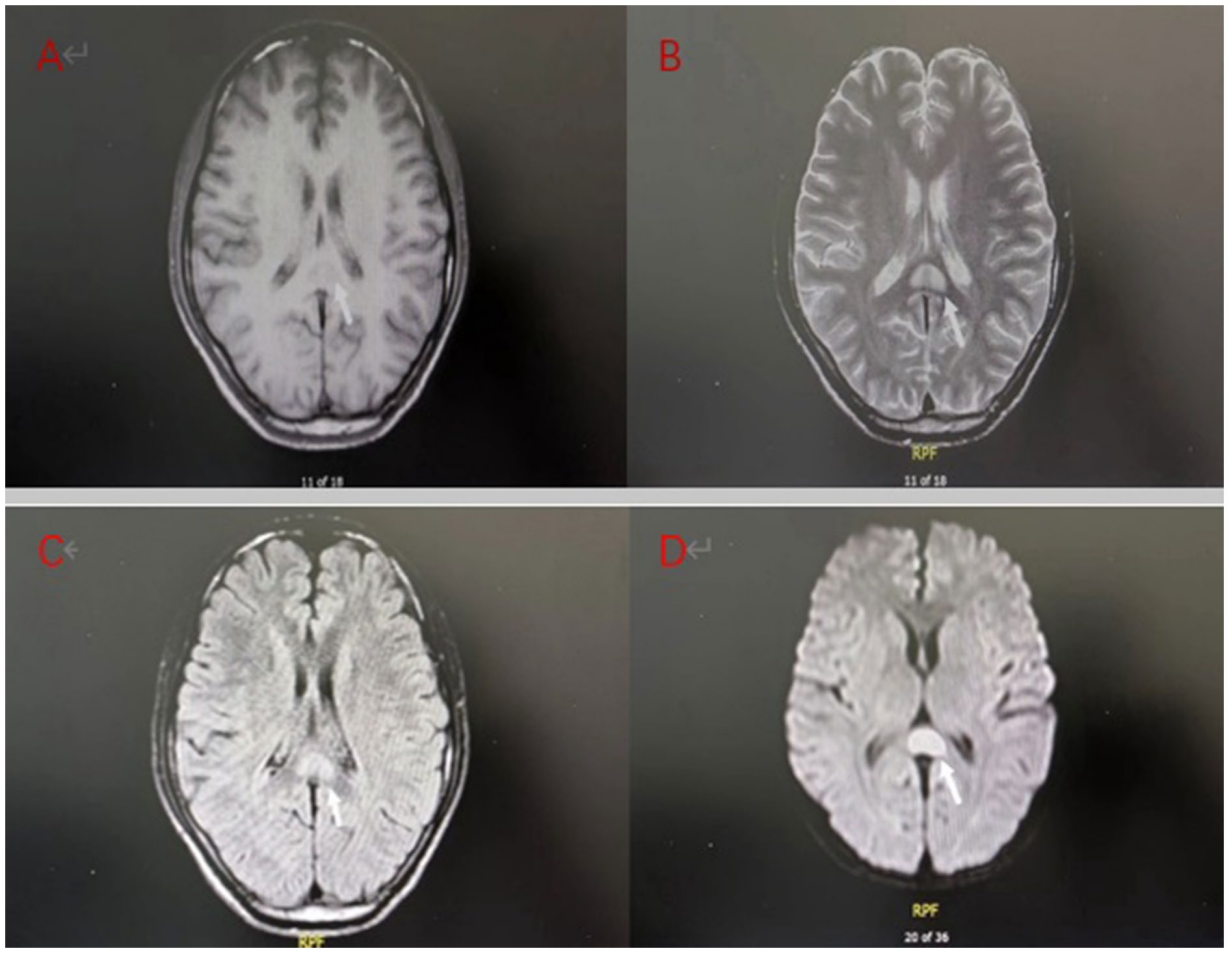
Brain MRI on admission of the patient: (A) T1 weighted imaging (T1WI) showed low signal intensity; (B) T2 weighted imaging (T2WI) showed high signal intensity; (C) Fluid attenuated inversion recovery (FLAIR) showed a high signal; (D) Diffusion weighted imaging (DWI) showed more pronounced hyperintensity than T2WI.
